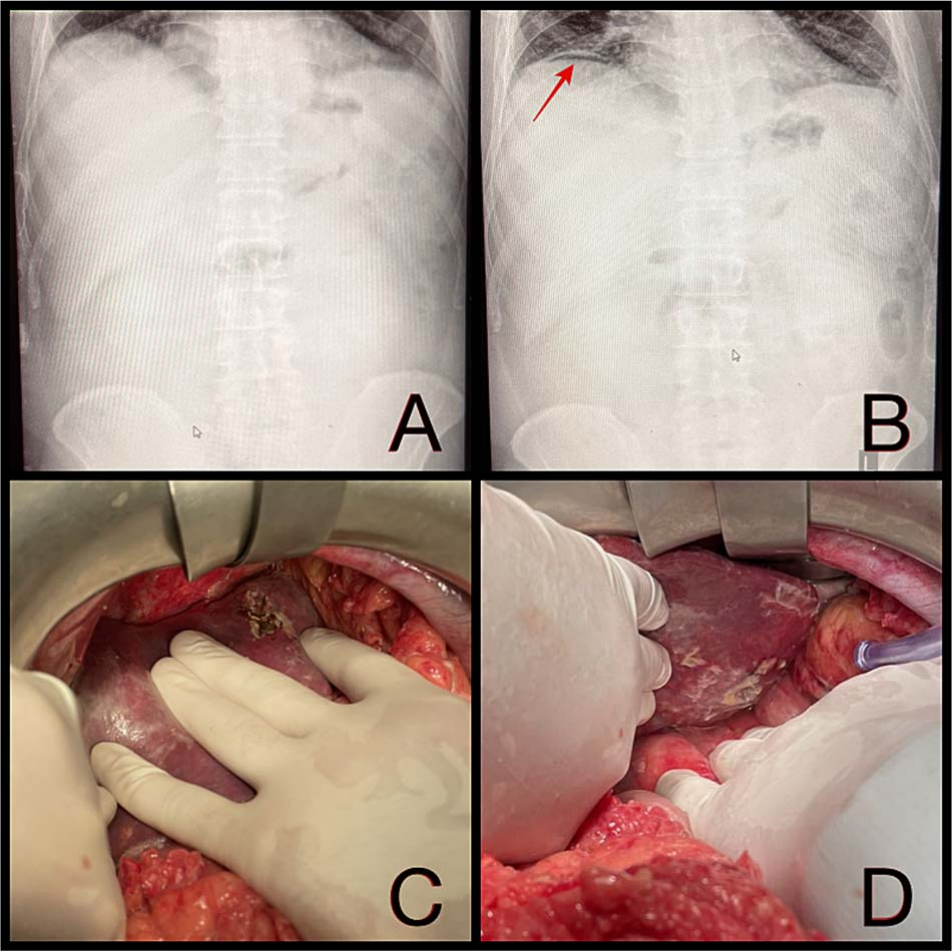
(A and B) Abdominal radiographs showing progression from no free gas (A) to a crescent of pneumoperitoneum beneath the right hemidiaphragm (B, arrow) within 2 hr. (C and D) Intraoperative findings of a ruptured pyogenic liver abscess in segment II, perforating both the diaphragmatic and visceral hepatic surfaces.
